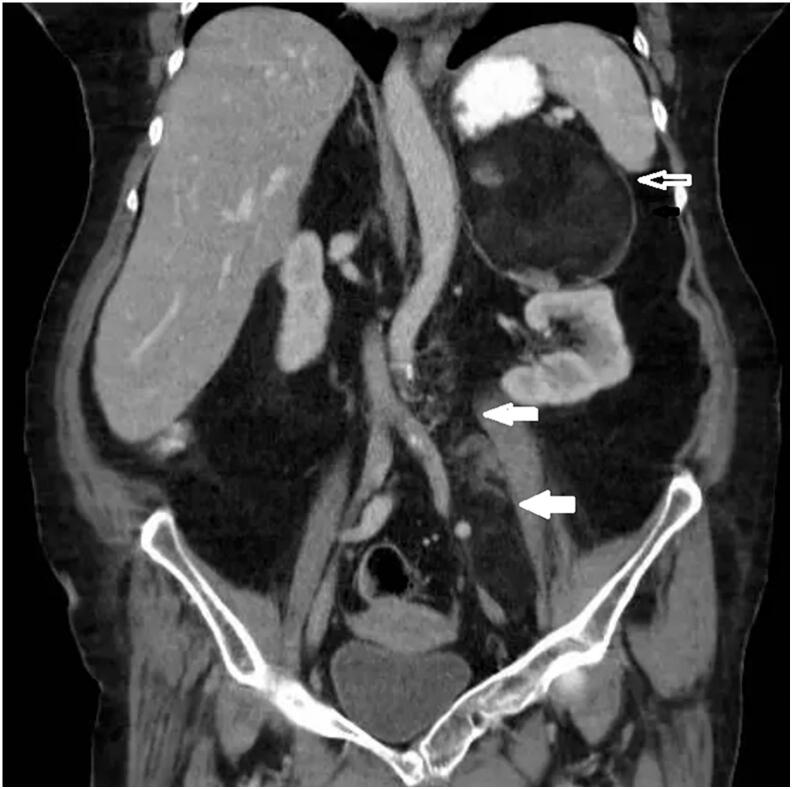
Coronal CECT (contrast-enhanced computed tomography) of the abdomen: the image reveals a round left adrenal mass with primarily fatty composition and soft tissue elements, indicative of myelolipoma (hollow arrow). Additionally, a lesion exhibiting fat density with soft tissue elements is observed in the para-aortic area, extending downward into the pelvic cavity near the left iliac vessels, raising suspicion for liposarcoma (solid arrows).
